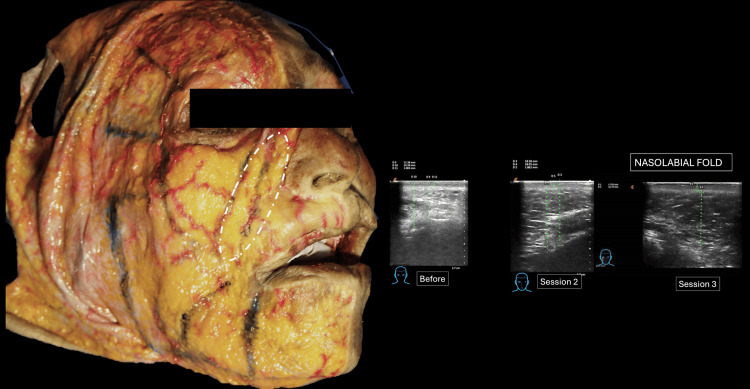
Comparative analysis of the ultrasound assessment with the anatomical component that locates the treated area. The oval shape is defined as the limits of the nasolabial panniculus. Latin American Center for Medical Training and Research (CLEMI), Bogota, November 2025.
